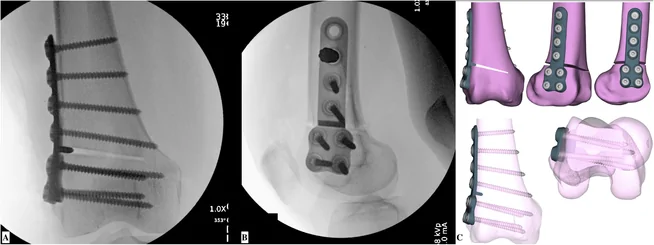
(A‐C) Final radiographic plate position with 3D rendering. Right knee, anteroposterior (A) and lateral (B) fluoroscopic images taken with patient in supine position. The position of the plate following fixation on anteroposterior (A) and lateral (B) fluoroscopic images can be compared with the preoperative projections as determined by the BodyCad (Quebec City, CA) software's 3D renderings (C). (3D, 3‐dimensional.)
